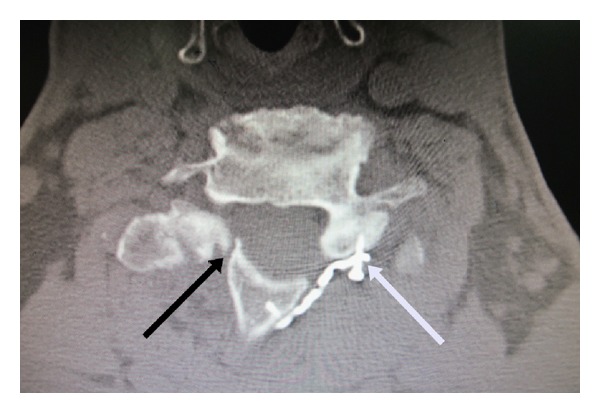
Axial CT scan at 6 months demonstrates screw back out (white arrow) and nonunion on the hinge side (black arrow) (no bone bridge at both dorsal and ventral cortices). There was no reclosure of laminoplasty.
